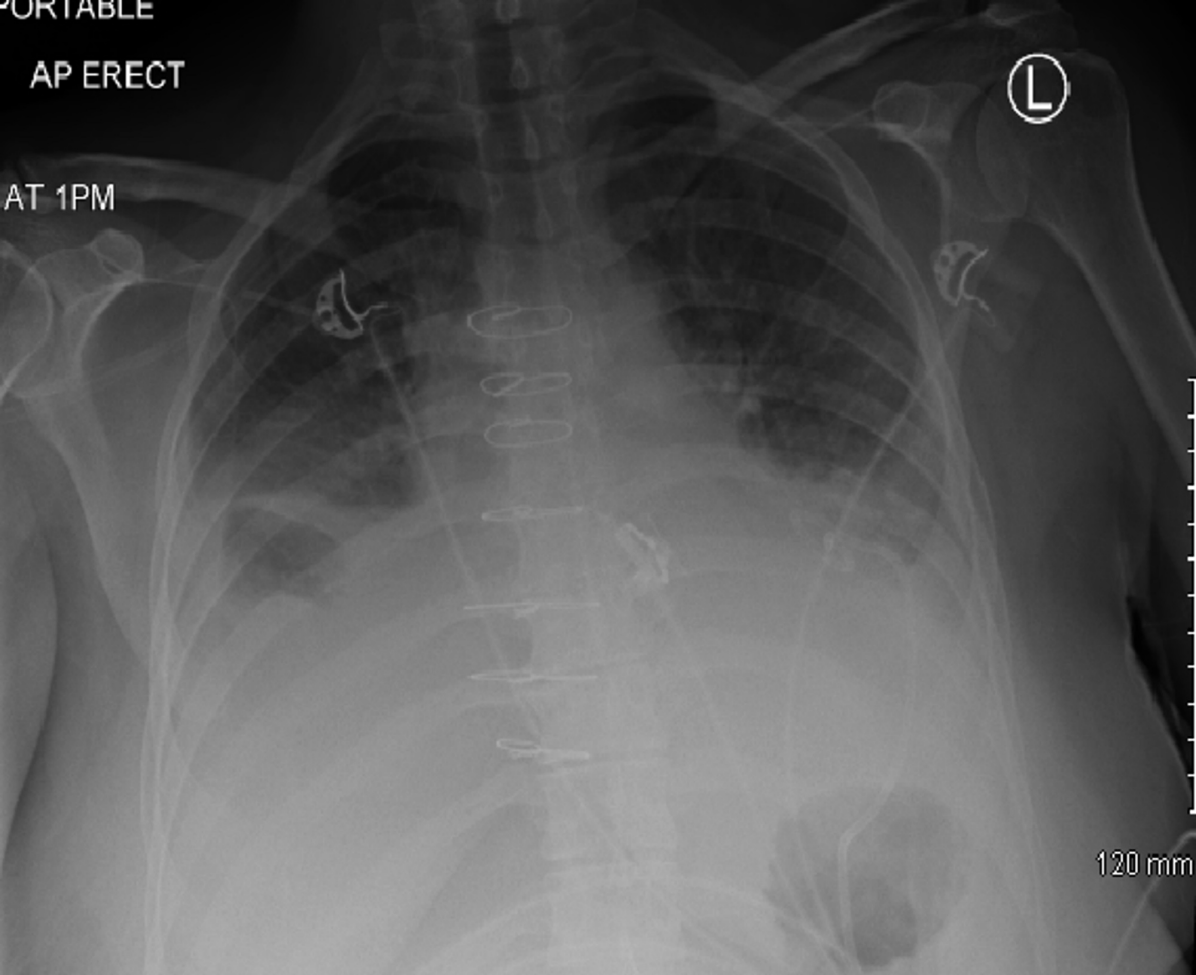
Chest X-ray (CXR) showing cardiomegaly and bilateral plural effusion
